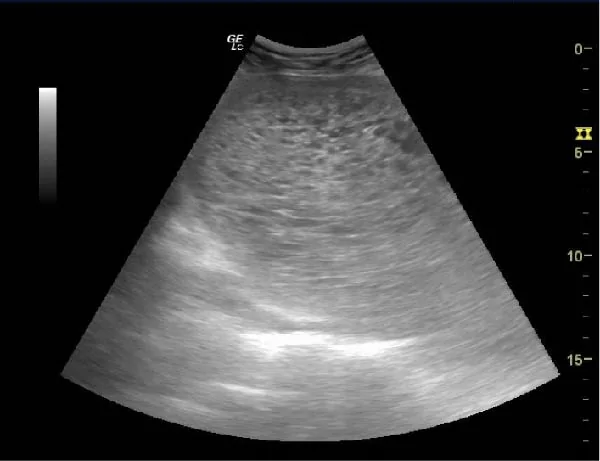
Transvaginal ultrasound with evidence of hydatidiform mole, just before the first curettage.
